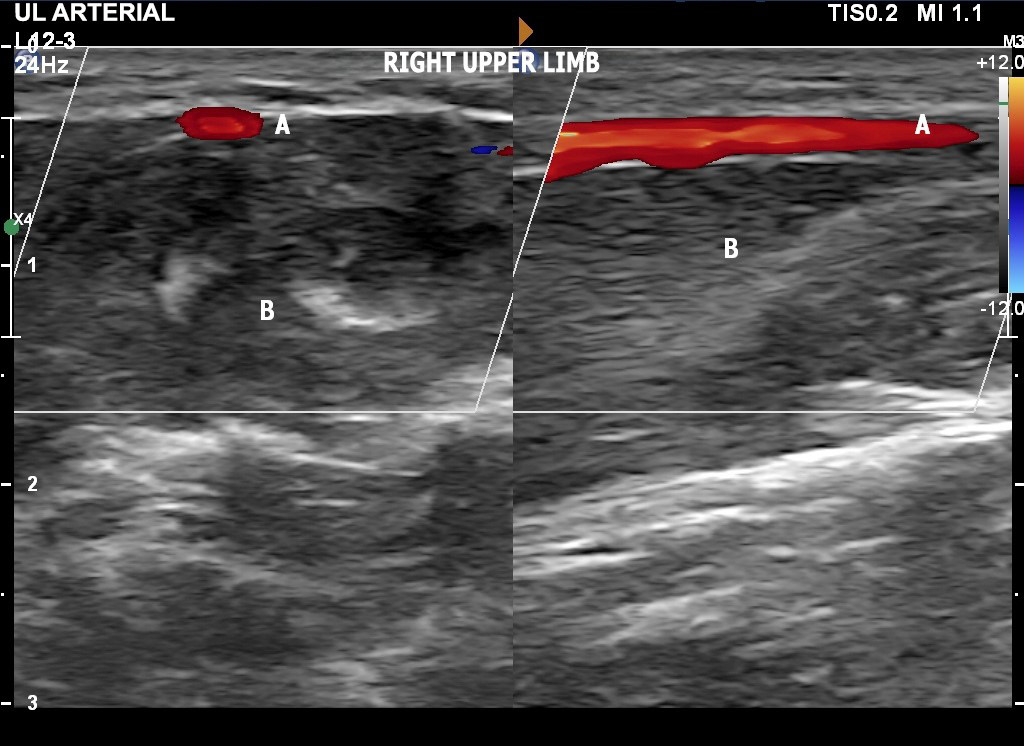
Doppler ultrasonography of the right upper limb showing presence of the superficial ulnar artery anatomic variant, showing the transverse image on the left and the longitudinal image on the right. Labels indicate (A) the ulnar artery, in a superficial position, and (B) the flexor muscles of the forearm.
